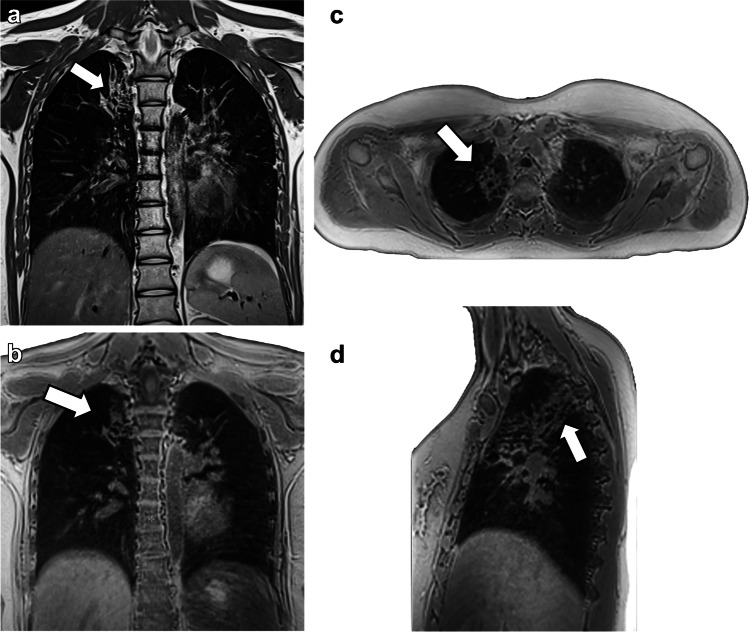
Magnetic resonance images in a 16-year-old girl with bronchiectasis in the upper lobes (*arrows*). a Coronal respiratory-triggered T2 fast spin echo and self-gated ultra-short echo time sequences with (b) coronal, (c) axial and (d) sagittal multiplanar reconstructions. Please note that air trapping distal to the dilated bronchi is only depicted in the latter
